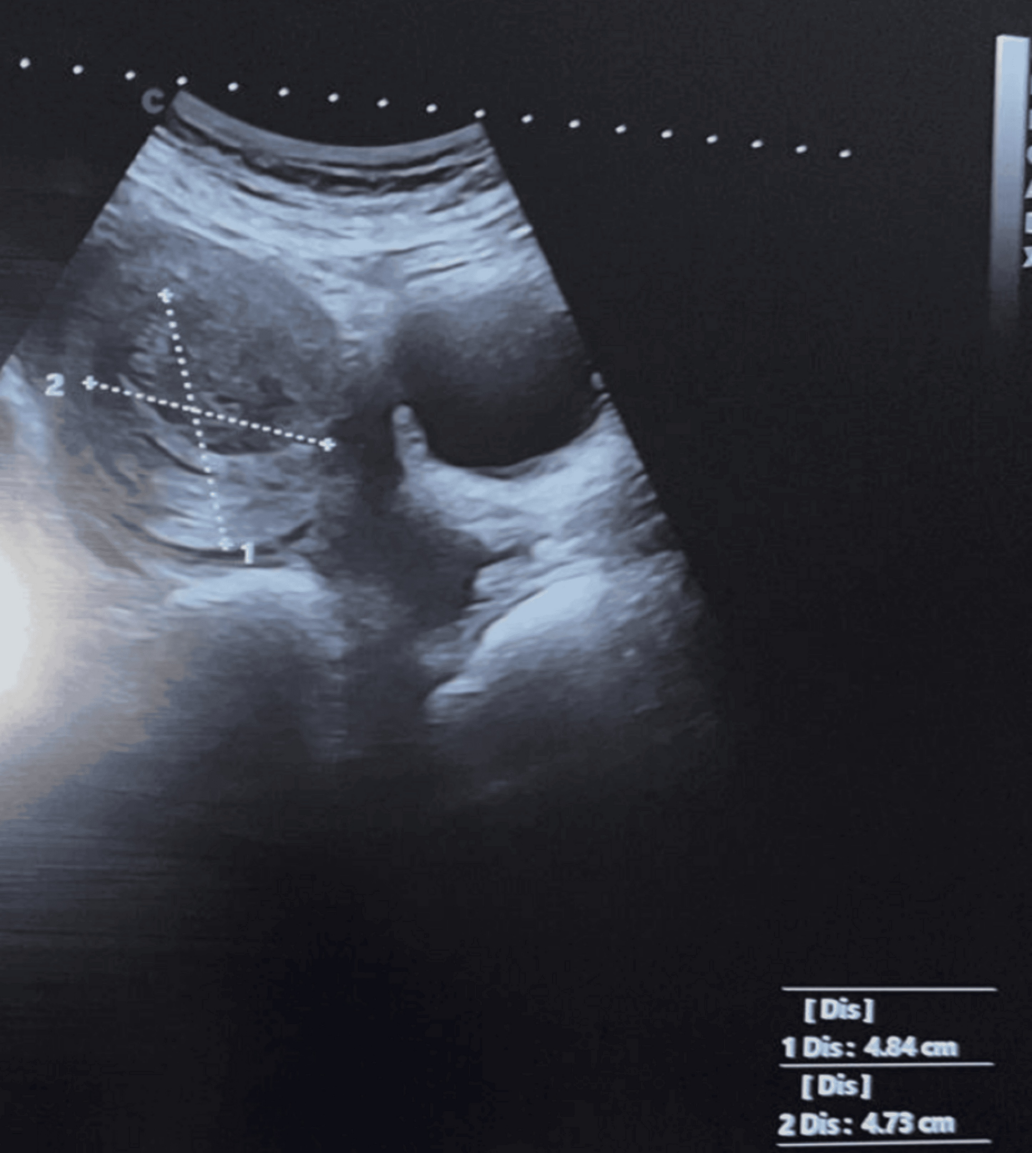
Pelvic ultrasound showing FIGO type 6 pedunculated fibroid measuring 4.8 × 4.7 cm at the fundus. FIGO, International Federation of Gynecology and Obstetrics
